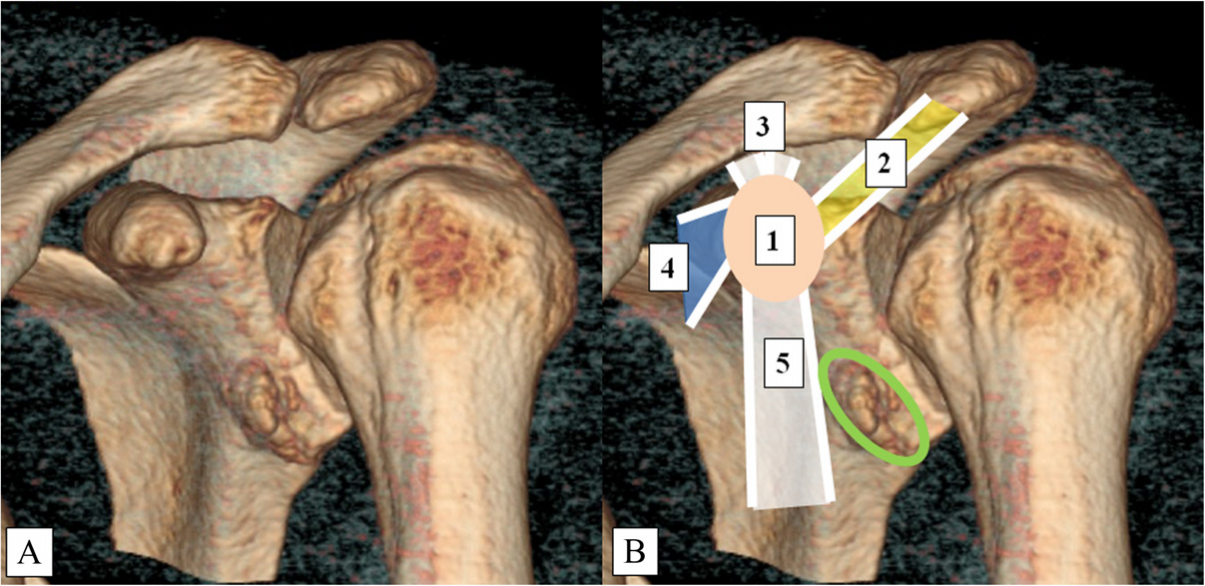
Three-Dimensional Reconstruction of a Computed Tomography Scan of Patient 1 (a, b). Landmark structures are highlighted (b). 1 (beige): CP; 2 (yellow): CAL; 3 (white): CCLs; 4 (blue): PMM; 5 (white): conjoined tendon. The green circle shows the bone defect of the anterior glenoid. CAL, coracoacromial ligament; CCL, coracoclavicular ligament; CP, coracoid process; PMM, pectoralis minor muscle
